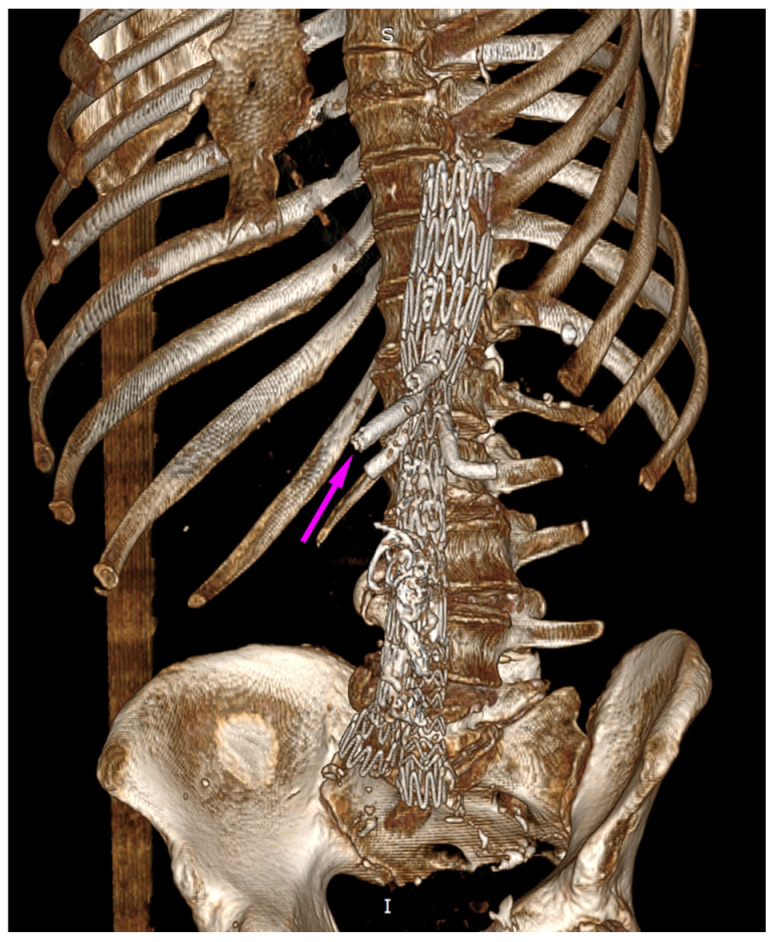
Post-intervention final effect. Newly implanted BeGraft Plus in a previously fractured BeGraft in the SMA. The visible image of the “stent within a stent” is marked with an arrow. Control CT—3D reconstruction.
